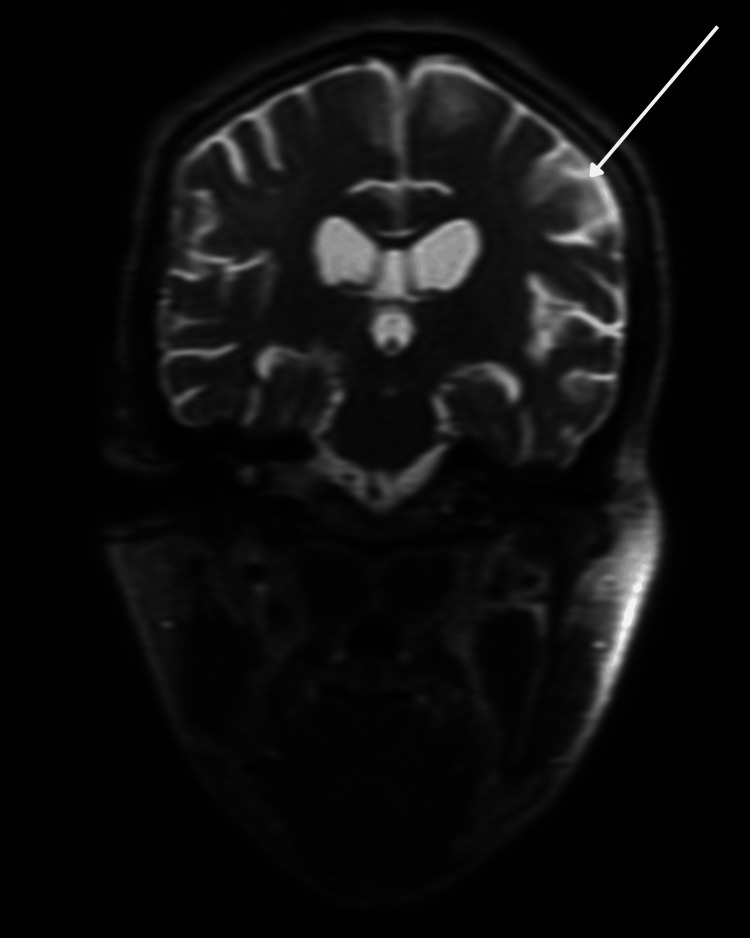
Coronal T2/FLAIR MRI demonstrating diffuse symmetric periventricular and deep white matter hyperintensity with subtle involvement of the bilateral gangliocapsular regions (arrow). FLAIR: fluid-attenuated inversion recovery
